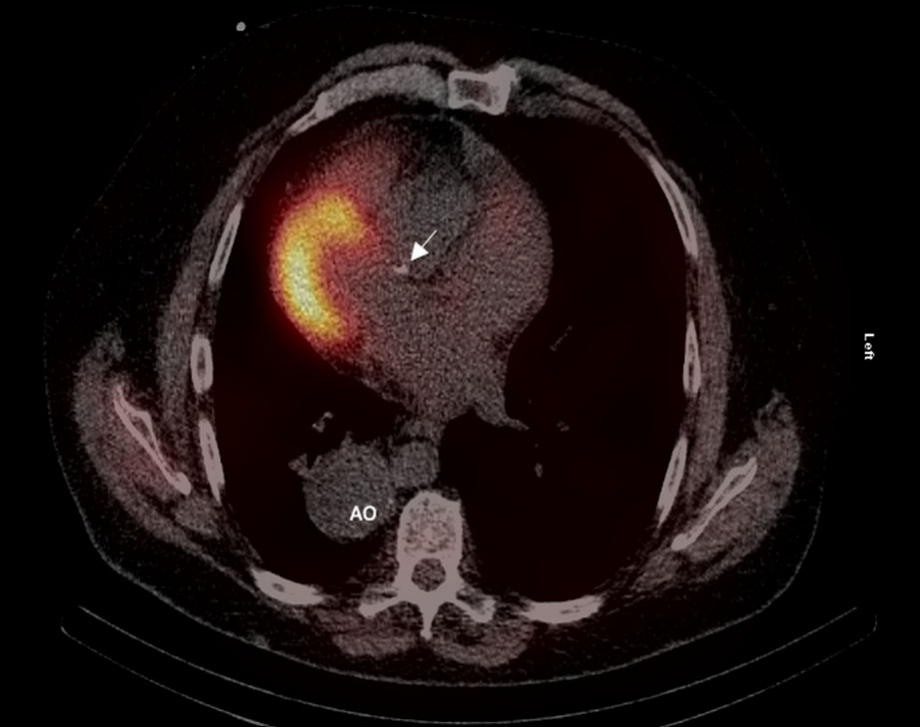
SPECT/CT of the patient demonstrating dextrocardia with situs inversus. A calcified plaque in the proximal left anterior descending artery is indicated by an arrow. The thoracic aorta is slightly dilated
